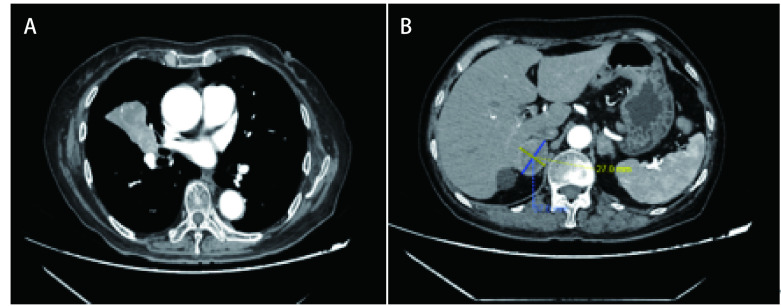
维持治疗期间出现肺部、肾上腺寡进展。A：胸部CT示肺门病灶较前增大；B：腹部CT示肾上腺转移较前增大。 Lung and adrenal metastases during maintenance treatment. A: chest CT showed hilar lesions larger than before; B: abdominal CT showed adrenal metastases larger than before. CT: computed tomography.
